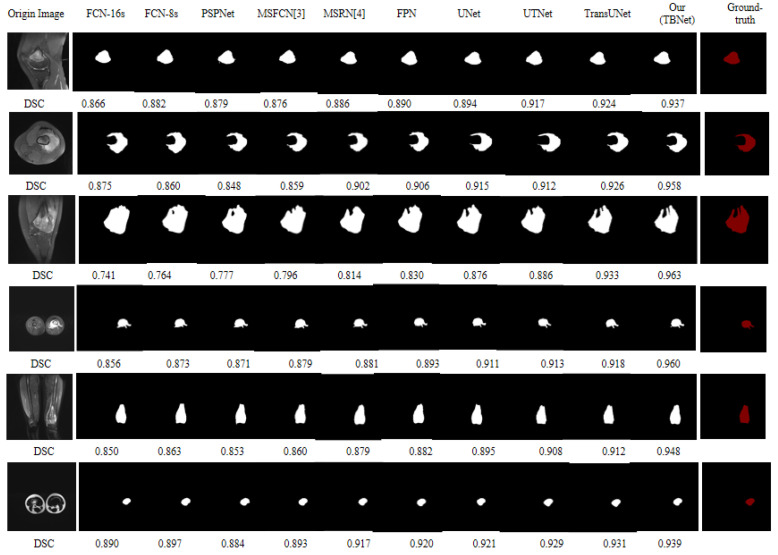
Comparison of different models for the segmentation of MRI images of osteosarcoma.
